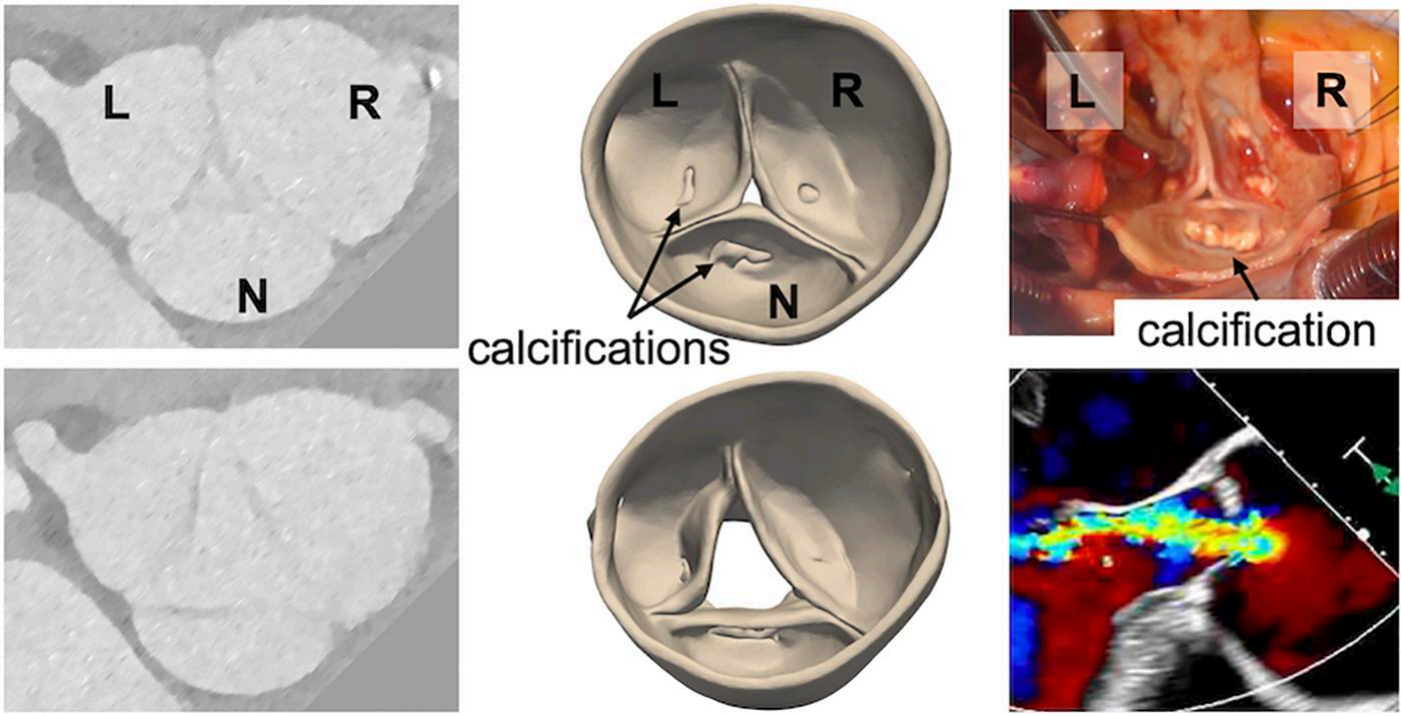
A severely regurgitant aortic valve that was suspected to be bicuspid but observed to be trileaflet on direct intraoperative inspection. (Left) Grayscale images from the pre-operative CT, (center) 3D models of the aortic valve obtained by CT segmentation, (right) direct visualization of the aortic valve and pre-operative transesophageal echocardiogram showing the regurgitant jet.
